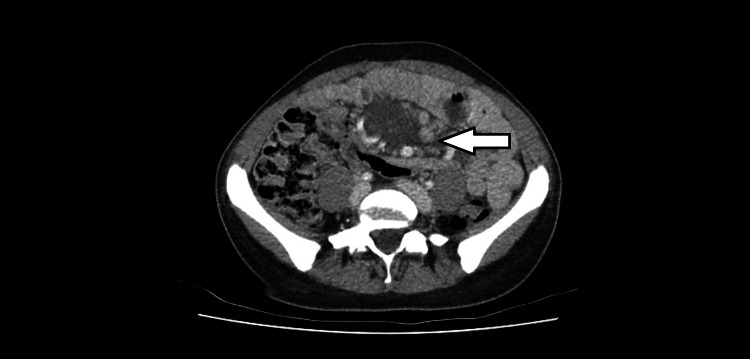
An axial abdominal CT scan image showing the mass closely related to a number of prominent vessels
